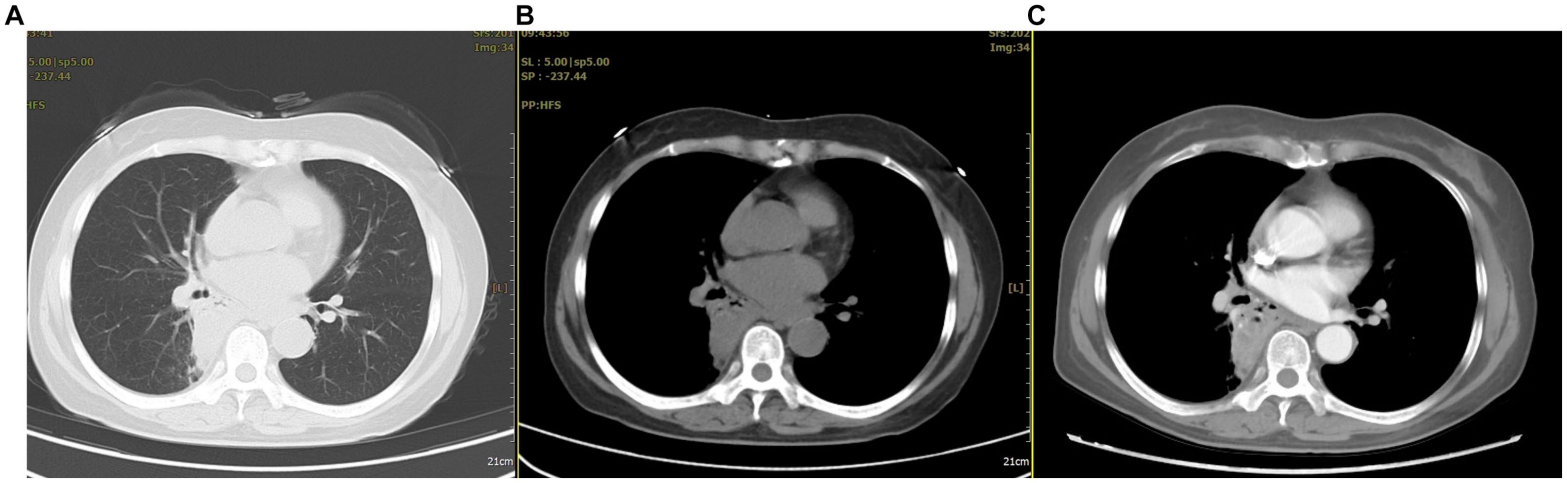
The chest CT revealed abnormal lesions in the lower lobe of the right lung. (A) In the lung window, a consolidation shadow was observed in the posterior segment of the right lower lobe. (B) In the mediastinal window, the lesion appeared to have regular margins. (C) After contrast enhancement, heterogeneous enhancement was noted within the lesion.
